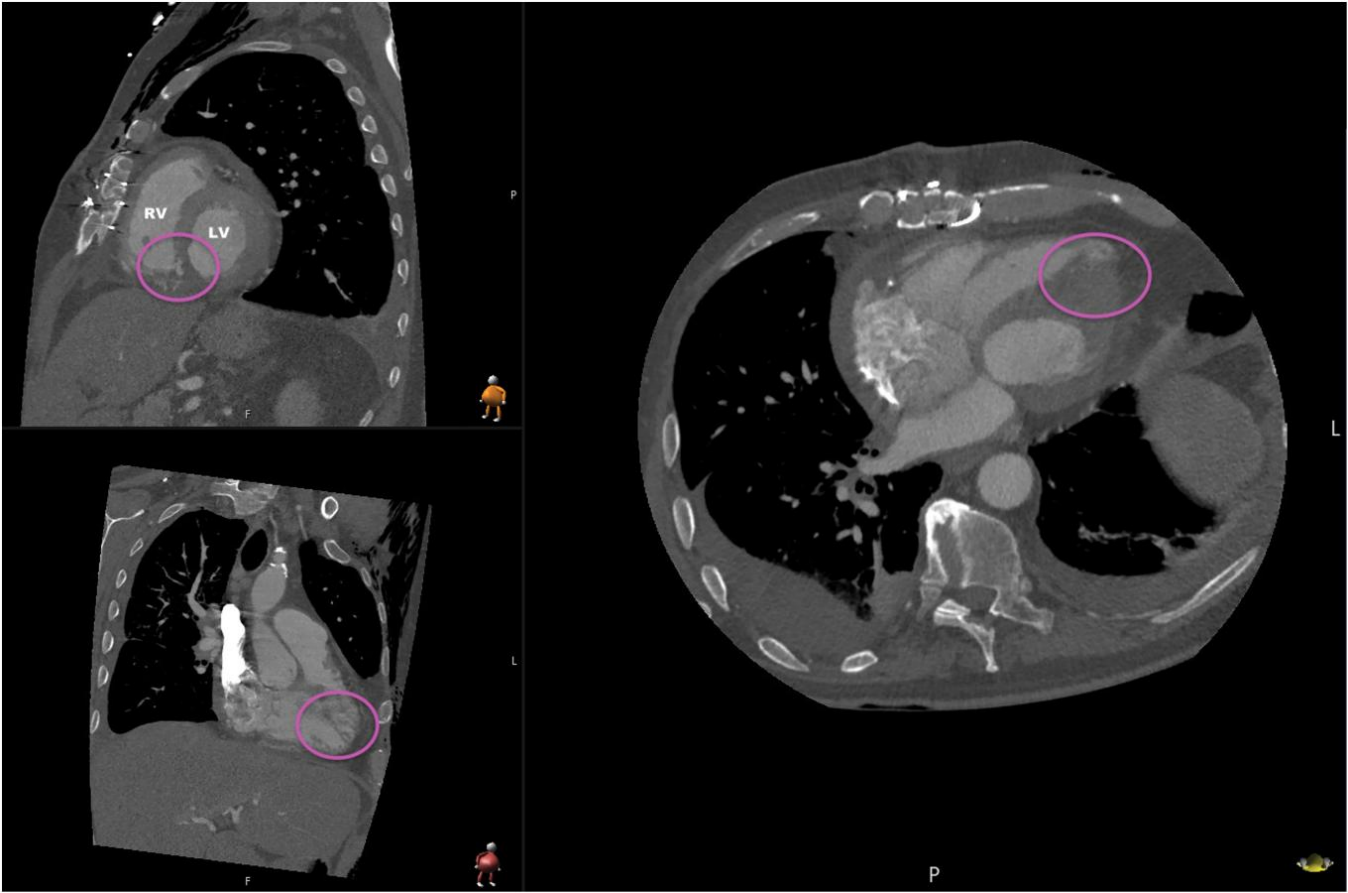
Post-operative computed tomography shows the triple patch with infarct exclusion (arrow) without residual shunt.
